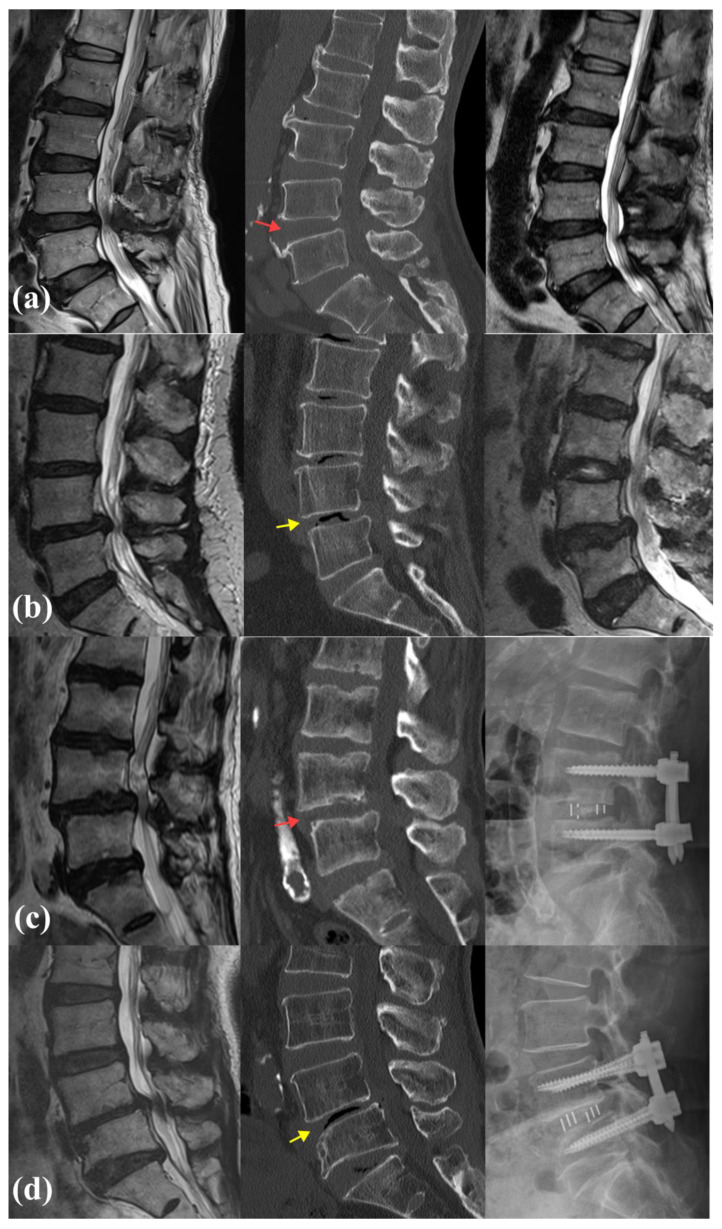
Comparative cases of patients with and without the VP undergoing endoscopic decompression and OLIF. (a) Preoperative MRI shows severe L4-5 stenosis with no VP observed on CT (non-VP, indicated by red arrow). Postoperative MRI confirms adequate decompression following endoscopic decompression. (b) Preoperative MRI shows severe L4-5 stenosis, with Grade 2 VP detected on CT (VP sign indicated by yellow arrow). Postoperative MRI confirms adequate decompression following endoscopic decompression. (c) Preoperative MRI reveals severe stenosis at L4-5 due to degenerative spondylolisthesis (DSL), but no significant VP sign is observed on CT (non-VP, indicated by red arrow). MIS OLIF was performed at L4-5. (d) Preoperative MRI shows severe stenosis at L4-5, with Grade 2 VP confirmed on CT (VP sign indicated by yellow arrow). MIS OLIF was performed at L4-5.5.
